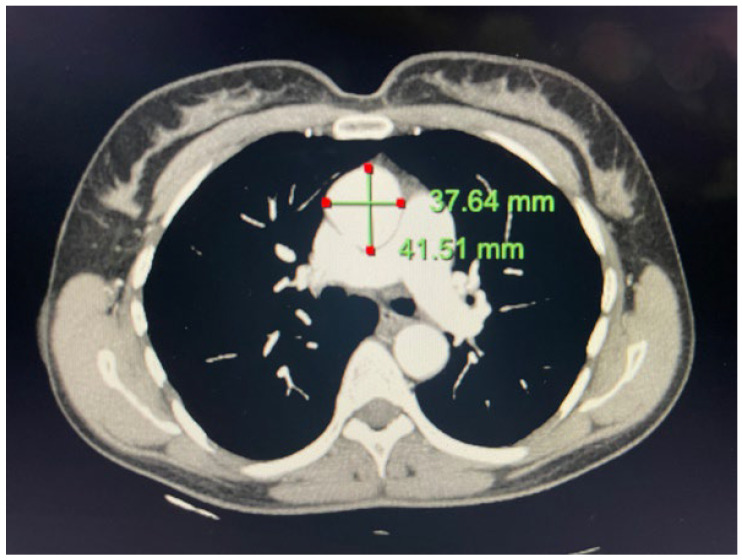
Aortic CTA with 2D axial slice demonstrating a 41.5 × 38 mm ascending aortic aneurysm.
